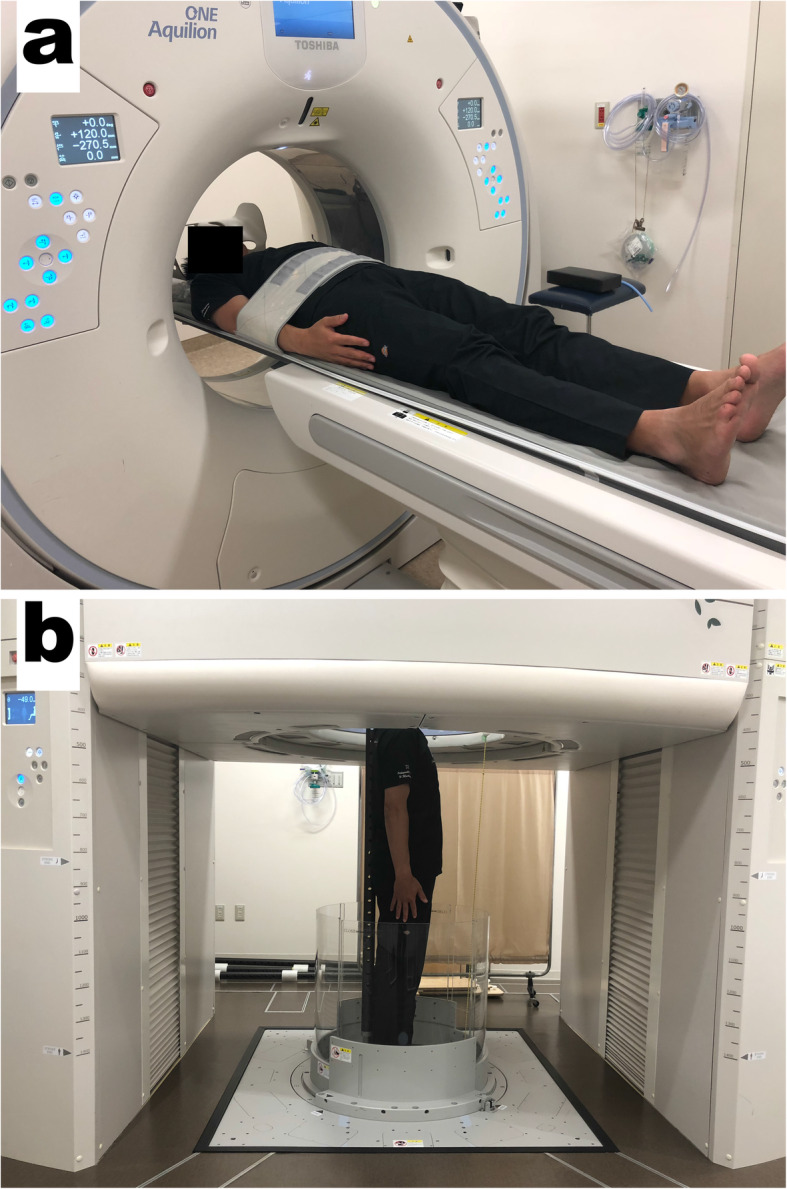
320-row conventional and upright computed tomography (CT) scanners. CT images of the bilateral shoulders were obtained with the shoulders adducted and the arms held in a neutral position, both in the supine position using a conventional scanner (a) and in the standing position using an upright scanner (b)
